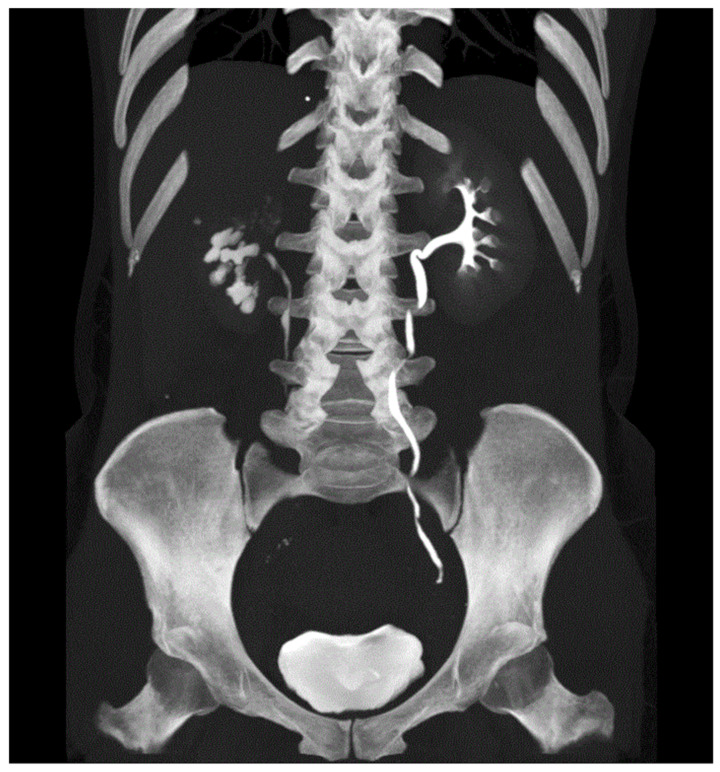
CT IVU showing right kidney delayed excretion and left ureter with multiple kinking and stenosis.
